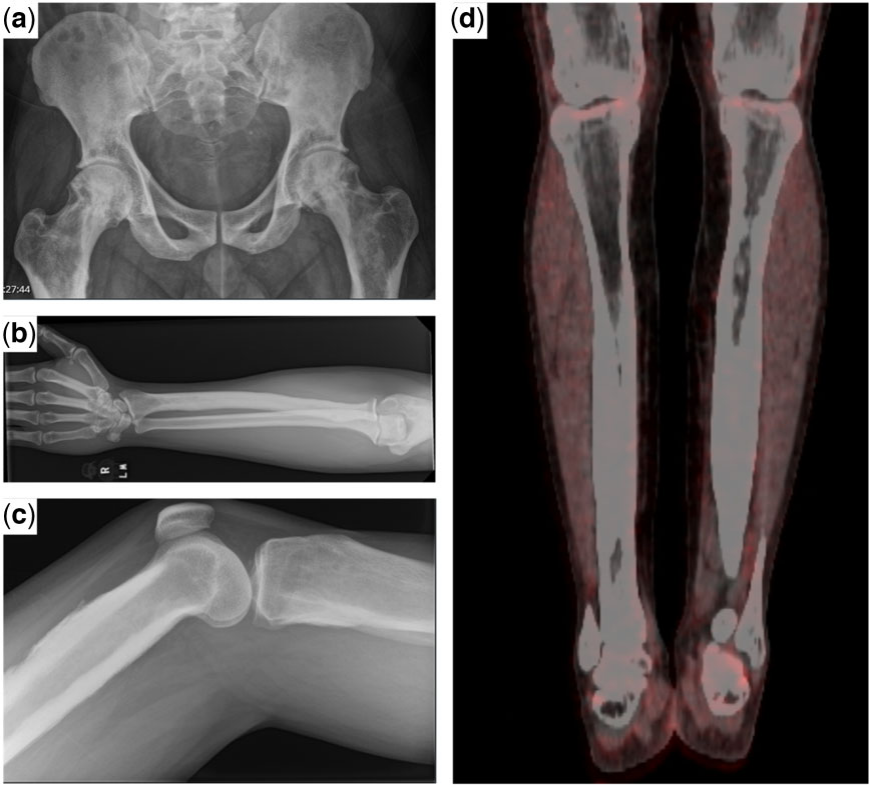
Imaging findings from the clinical case. Plain radiographs demonstrating osteosclerotic changes (panel a), with widespread diaphyseal dysplasia and cortical thickening in the long bones (panels b–c). Fluorodeoxyglucose (FDG)-PET images of the tibial bones demonstrate the symmetry of the changes, as well as the lack of FDG-avidity in the skeleton (panel d)
